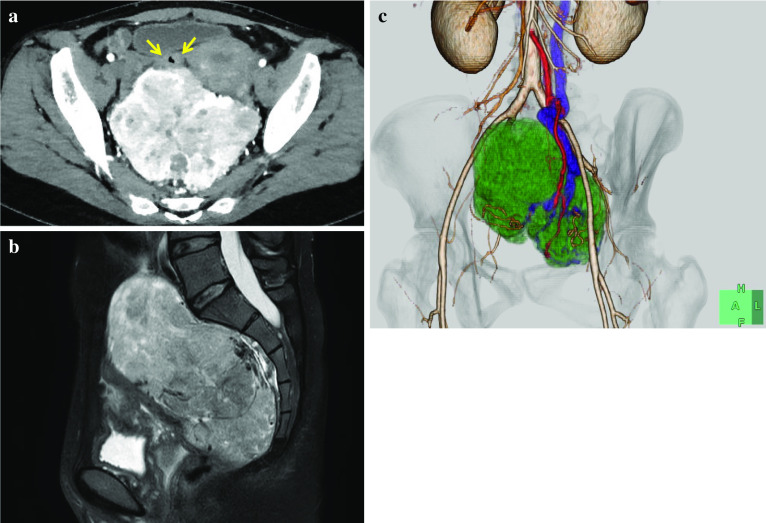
Computed tomography (CT) and magnetic resonance imaging (MRI). a Contrast-enhanced CT image showed a hypervascular mass occupying the pelvic cavity, displacing and compressing the rectum (yellow arrows). The tumor showed marked enhancement in a heterogeneous pattern. b T2-weighted pelvic MRI image showed a high-signal-intensity tumor in the pelvis. c 3D-CT image reconstruction showed the tumor occupying the pelvis (green), the tumor-feeding artery (the inferior mesenteric artery colored in red), and the tumor draining vein (the inferior mesenteric vein colored in blue)
